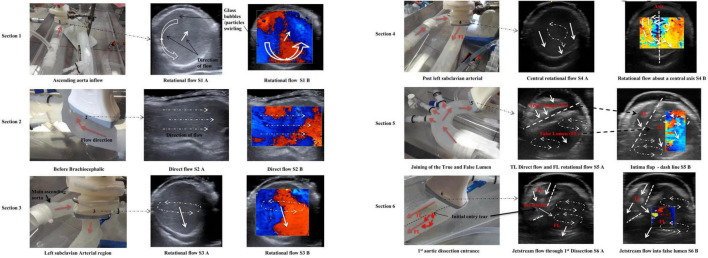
Monitored flow patterns by ultrasound for Sections 1–6.
